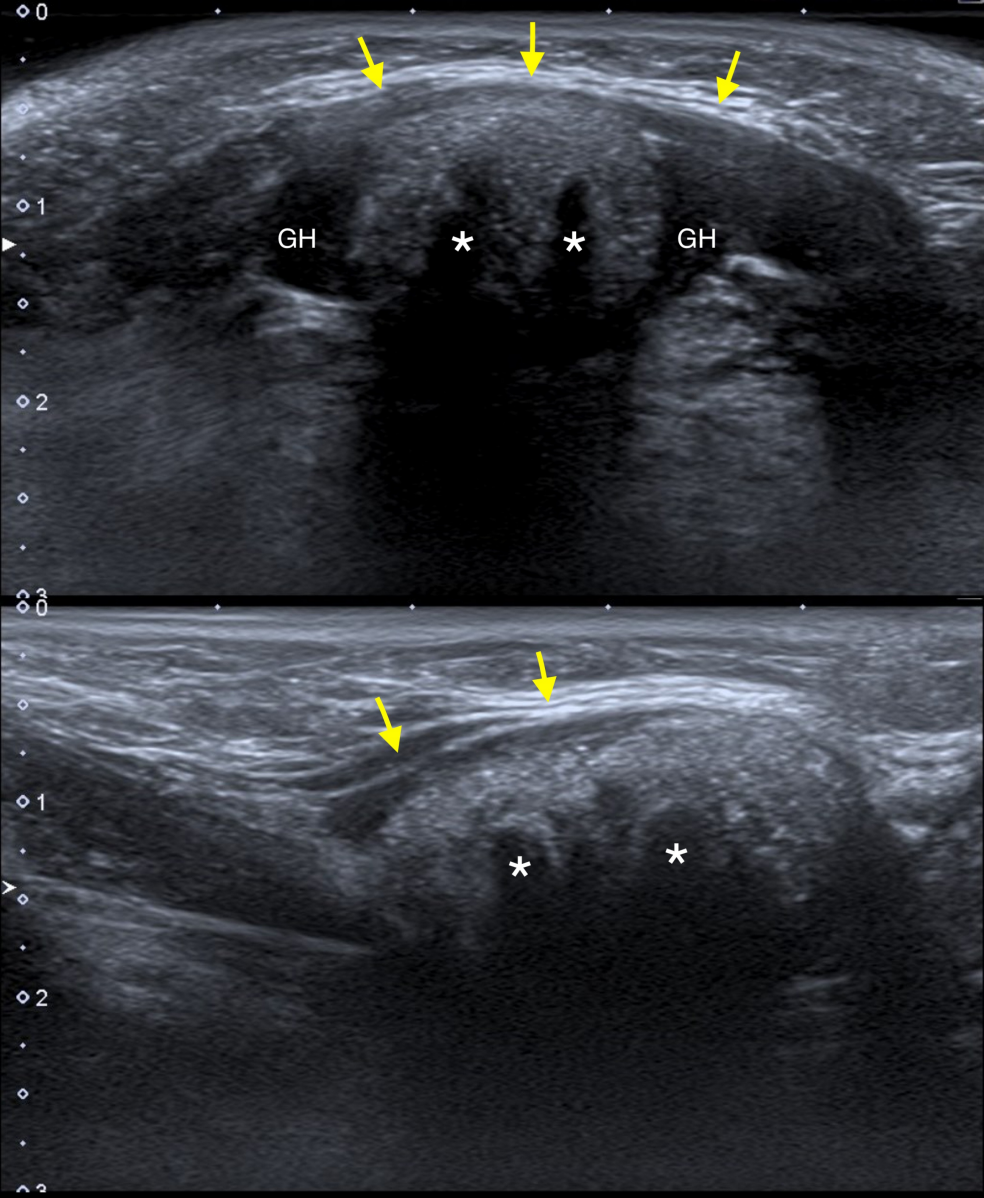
Submandibular ultrasonography Transverse (upper panel) and longitudinal (lower panel) scans show a hyperechoic lesion above the mylohyoid muscle (arrows) and between the geniohyoid muscles; highly reflective echoes with acoustic shadowing are appreciable (asterisks).
